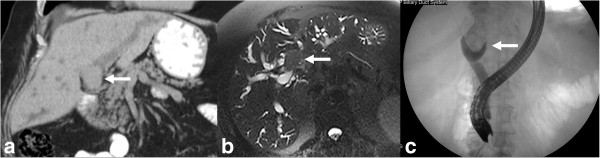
Hilar CCA presenting as an intraluminal mass with biliary obstruction. The mass is isodense (arrow) on the coronal non-contrast enhanced CT (a) and hypointense on axial T2-weighted image (b) with upstream dilatation of the intrahepatic ducts. ERCP (c) shows a filling defect (arrow) representing the mass extending into the common bile duct with no filling of the intrahepatic ducts.
